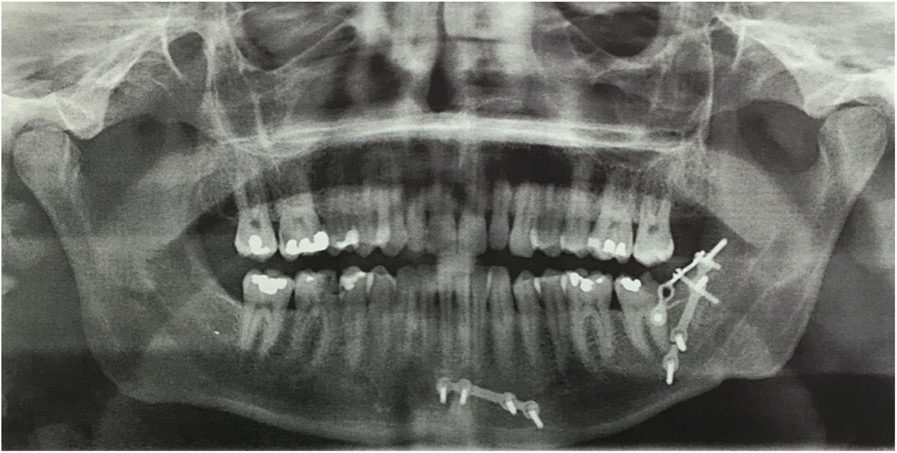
Preoperative panoramic X-ray. The trabecular bone of the region of teeth 33–37 is normal. No alteration of the periodontal ligament space is apparent. The inferior alveolar canal retains normal characteristics. Note the osteosynthesis plates in the paramedian and retromolar position of the left mandible
